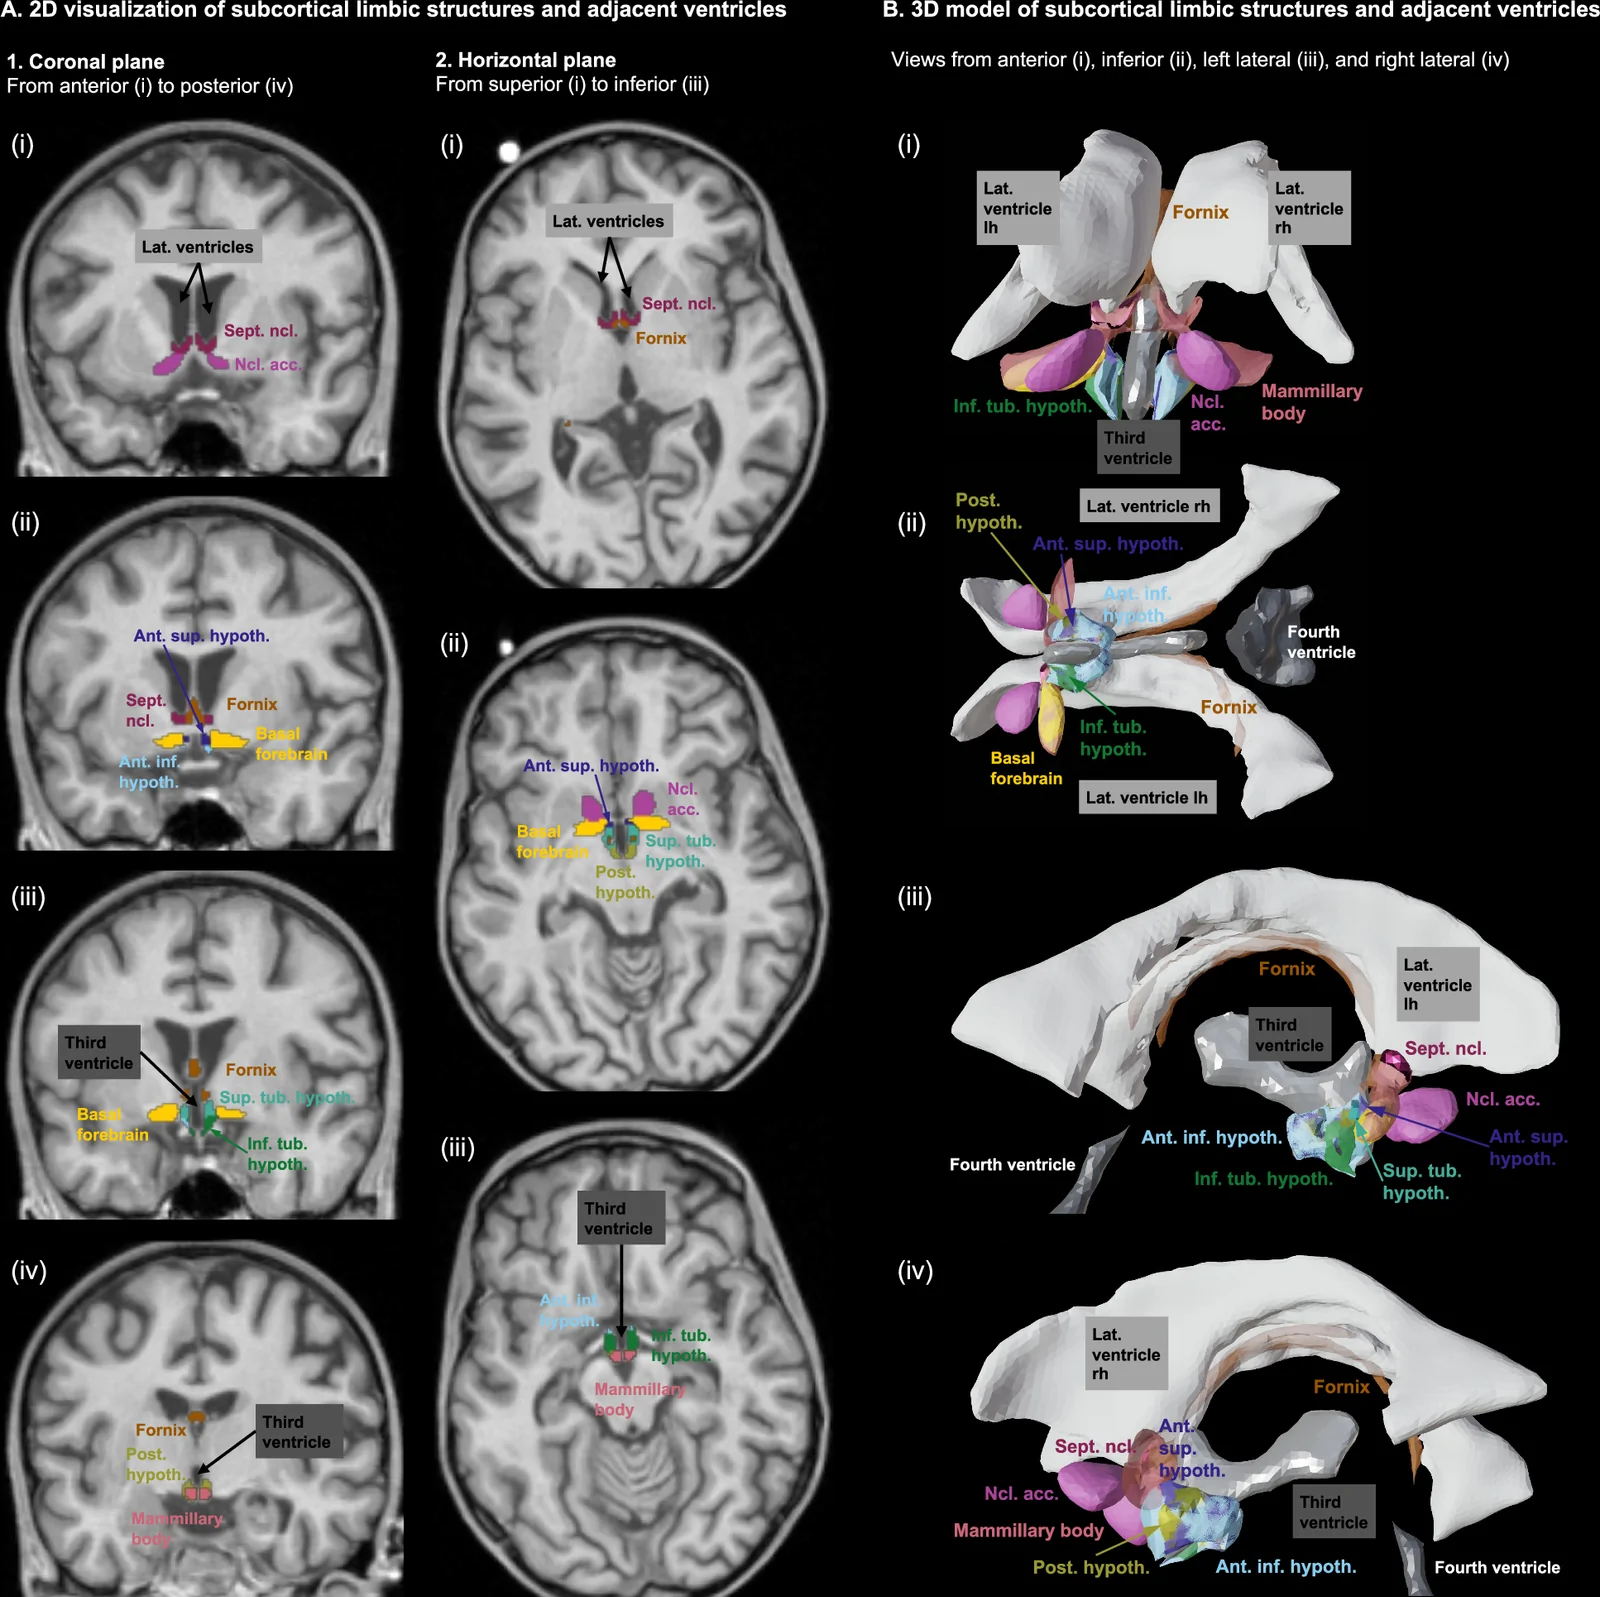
Two- and three-dimensional visualizations of subcortical limbic structures. Ant. inf. hypoth. anterior inferior hypothalamus, ant. sup. hypoth. anterior superior hypothalamus, inf. tub. hypoth. inferior tubular hypothalamus, lat. lateral (ventricle), lh left (brain) hemisphere, ncl. acc. nucleus accumbens, post. hypoth. posterior hypothalamus, rh right (brain) hemisphere, sept. ncl. septal nucleus, sup. tub. hypoth. superior tubular hypothalamus. MRI parameters (T1-weighted): MP-RAGE (magnetization prepared rapid acquisition gradient echo) sequence with 176 sagittal slices (1 mm thickness, no gap), TR = 1900 ms, TE = 2.26 ms, flip angle = 9˚, resolution = 1.0 × 1.0 × 1.0 mm^3^, FoV = 256 × 224 mm^2^, bandwidth = 200 Hz/pixel. A 2D FreeView [63] snapshots in different planes and B 3D model in different perspectives (created with Blender [108]) of the subcortical limbic structures analyzed in this study (and adjacent ventricles). A, B were obtained from a scan of a study participant after FreeSurfer version 7.1.1-based subcortical limbic segmentation [40] and hypothalamic subsegmentation [41]. To enhance accessibility, color blindness-friendly labeling was used in all figures (https://personal.sron.nl/~pault/data/colourschemes.pdf).
